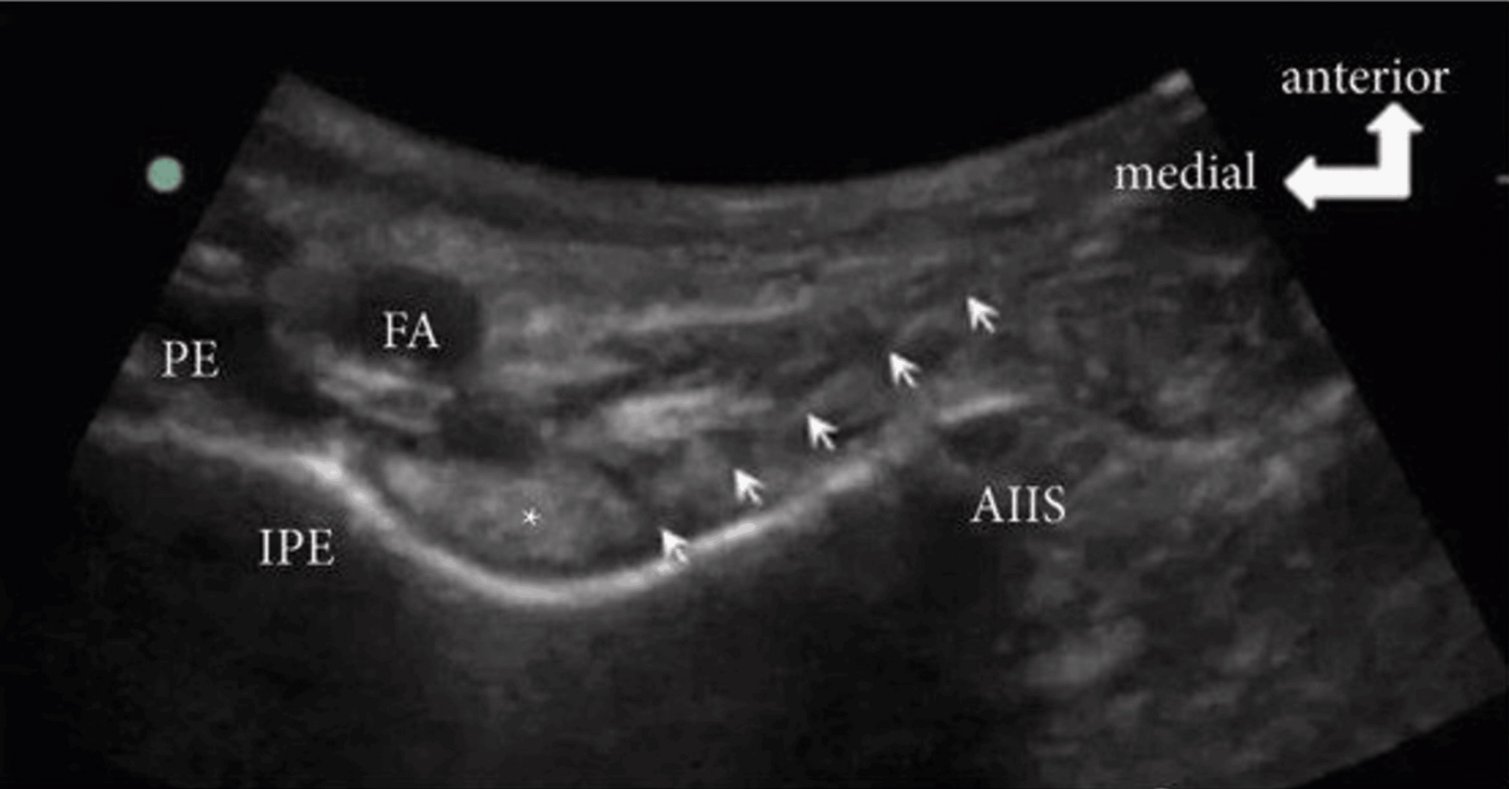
Ultrasound Image of PENG block FA: Femoral artery; PE: pectineus muscle; AIIS: anterior Inferior Iliac spine; IPE: iliopubic eminence
